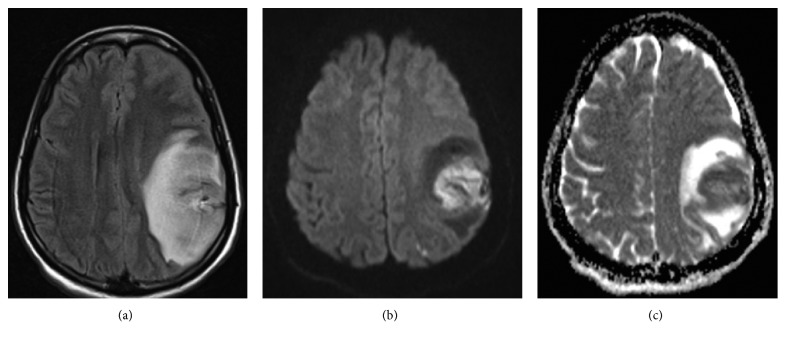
Axial FLAIR (a) representative image demonstrates large area of FLAIR hyperintensity involving the left frontoparietal region representing edema with mass effect, new/significantly increased compared to the prior study. There is associated restricted diffusion (as evidenced by DWI hyperintensity (b) and ADC hypointensity (c)) compatible with evolving acute infarct.
